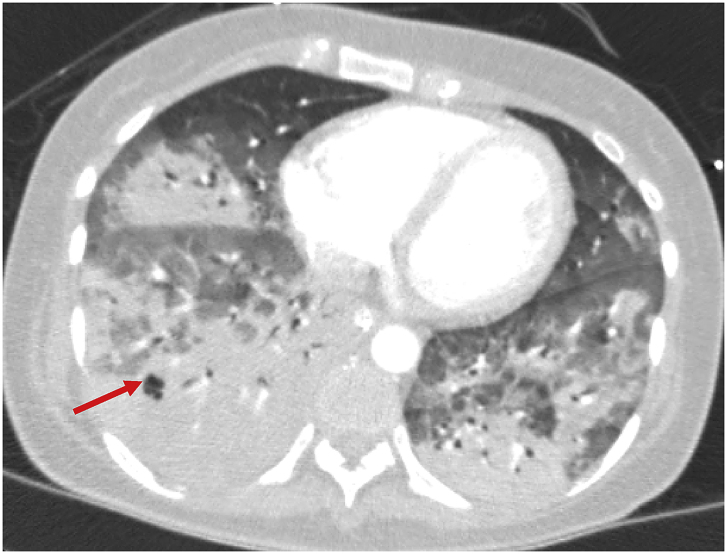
CT Chest on HD 5 showing multifocal nodular consolidations with early cavitation (red arrow).
